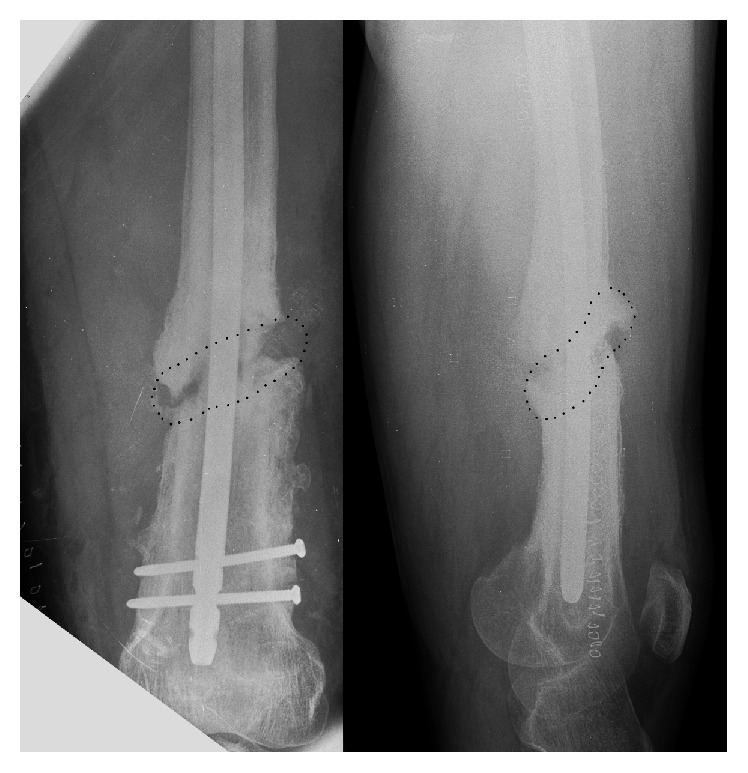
The femoral shaft fracture was stabilized with retrograde intramedullary nailing and the bone loss was restored with a corticoperiosteal pedicled flap. The X-ray shows less radiopacity of the flap (dotted line).
